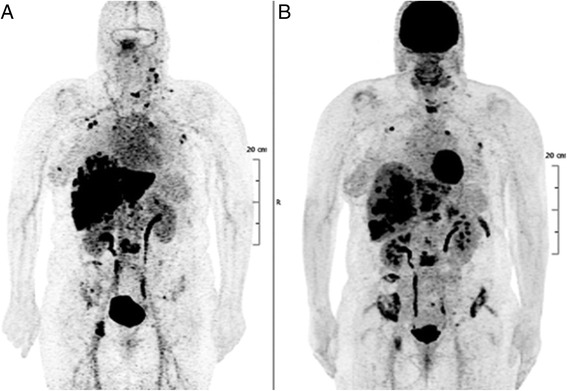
PET imaging of a patient with metastatic breast carcinoma. a Pretargeted immuno-PET performed using the TF2 anti-CEA bispecific antibody and the ^68^Ga-labelled IMP-288 peptide detects more lesions than FDG-PET (b)
